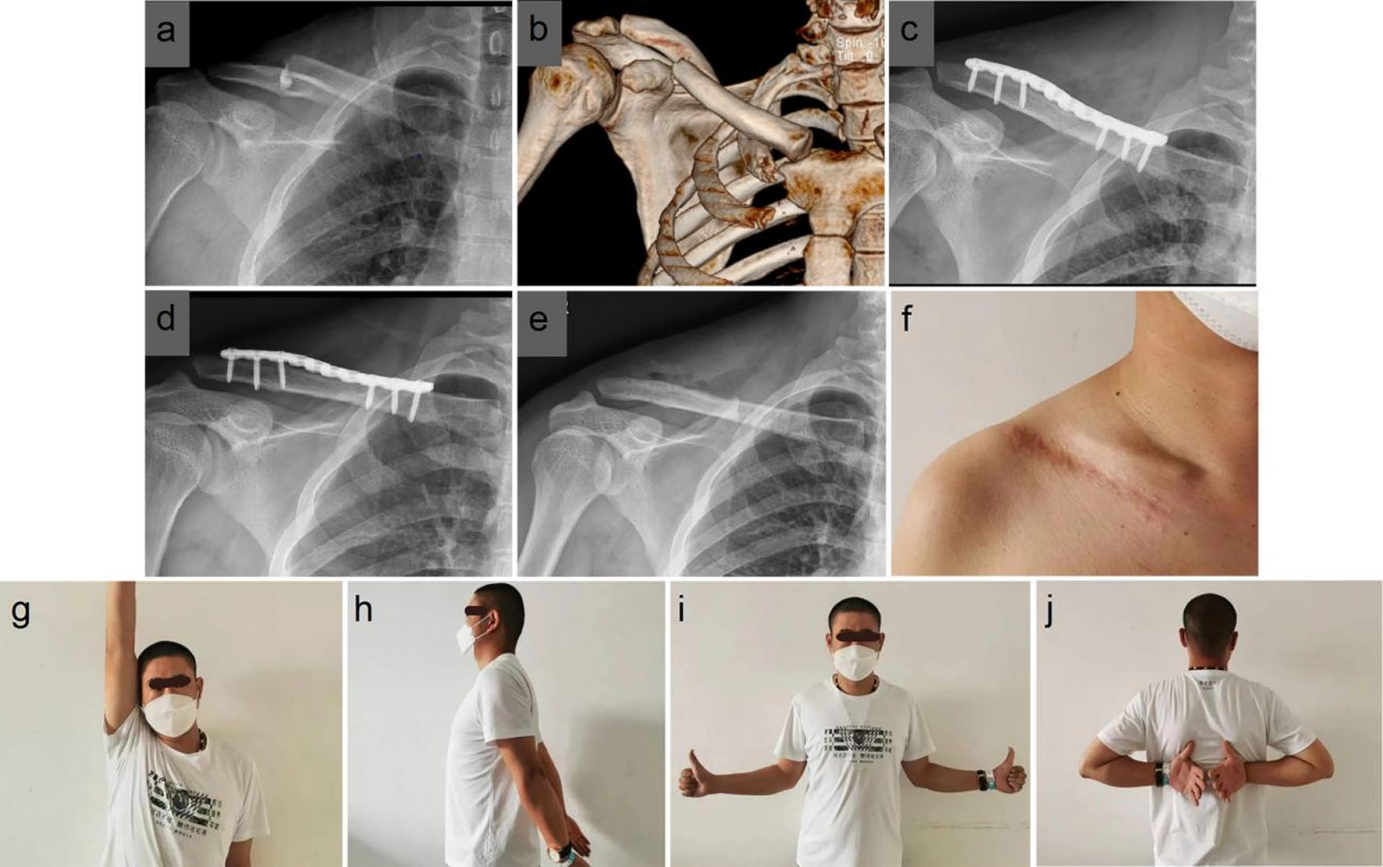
A 36-year-old male who sustained a fracture during a traffic accident. a, b Preoperative orthogonal X-ray and CT 3D reconstruction of the right shoulder showed a fracture of the middle clavicle. c Orthogonal X-ray of the right shoulder 2 d after surgery showed good fracture repositioning. d Orthogonal X-ray of the right shoulder 1 year after surgery showed good fracture healing. e Plain radiograph of the right shoulder 1 year after removal of the locking plate showed a basically normal clavicle morphology with bone loss at the nail path. f Skin scarring of the incision 1 year after surgery. g–j The patient's shoulder function had returned to normal at the 1-year-postoperative review
